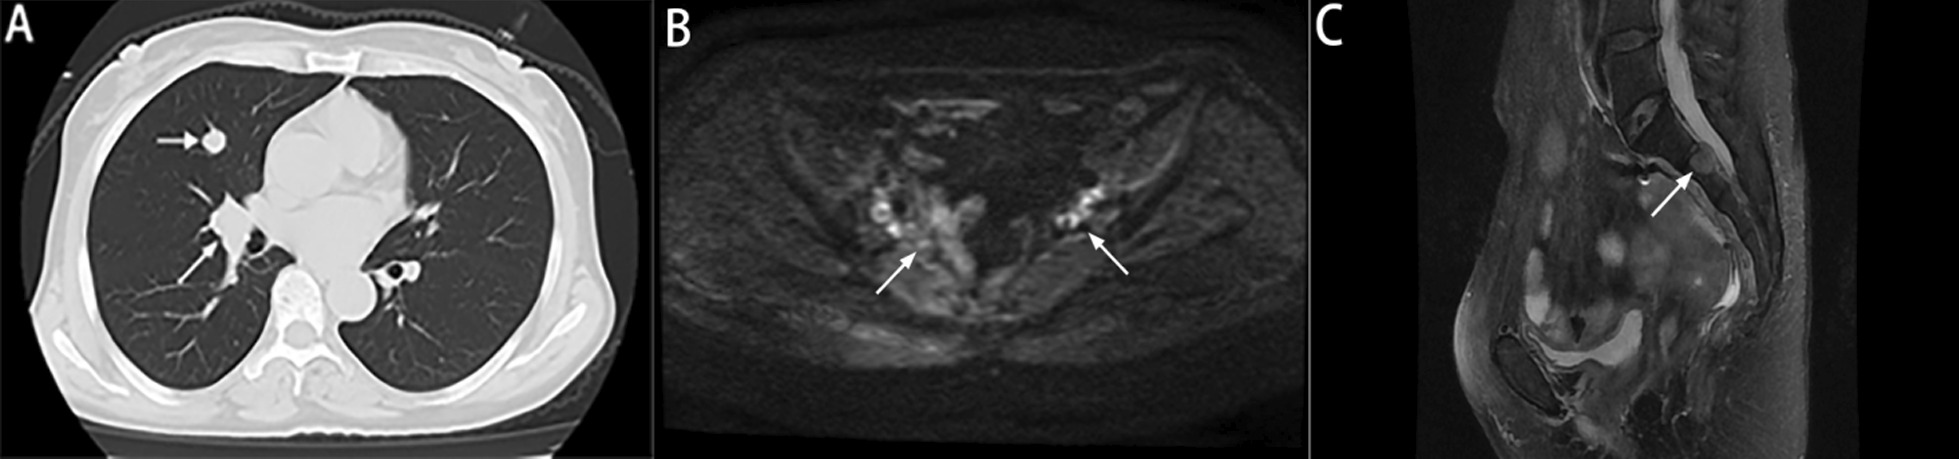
Imaging data from January 2022 A CT showed that the right pulmonary nodules had obviously progressed (arrow). B The pelvic nodules were significantly enlarged (arrow). C MRI showed sacral vertebral metastases (arrow)
